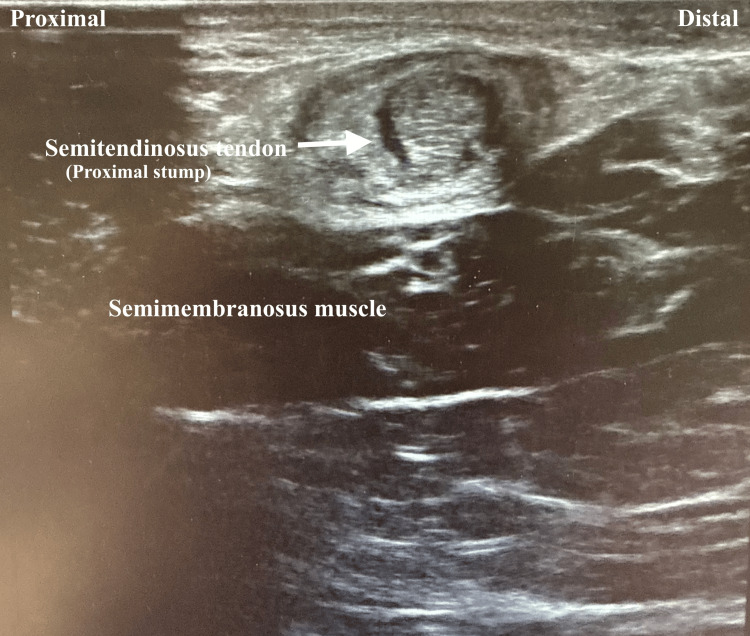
Short axis view of ultrasound of knee. The semitendinosus is showing a stump with surrounding fluid suggestive of tendon retraction (arrow).
